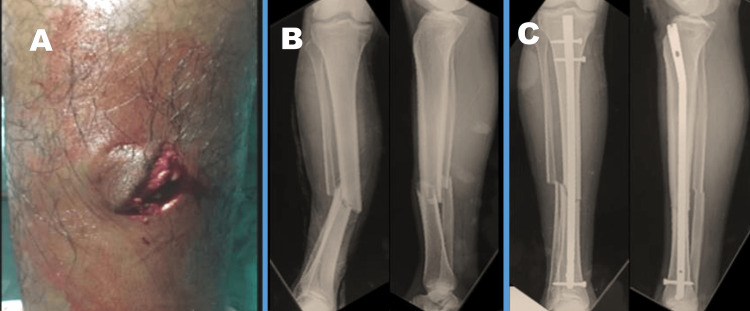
Open fracture of the lower limb. A: Gustilo-Anderson grade 2 fracture. B: Preoperative X-ray of the lower limb. C: Postoperative X-ray of the lower limb.
